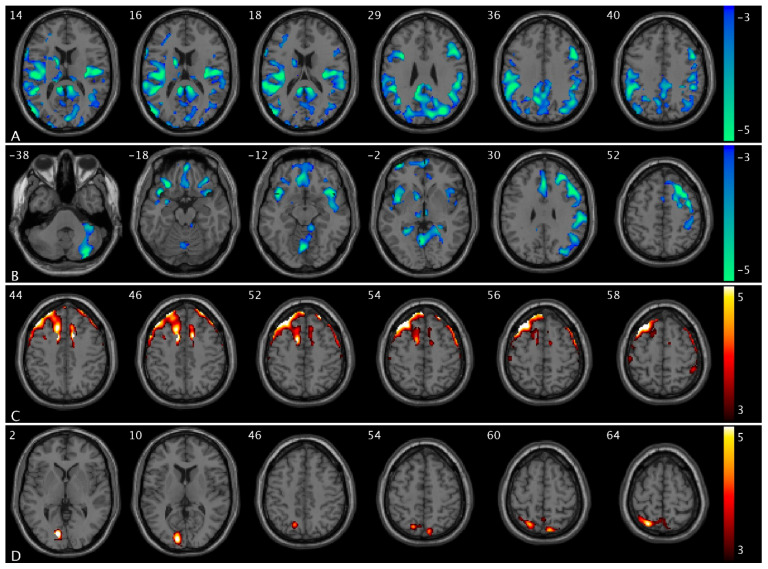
Statistical maps overlaid on T1-weighted images showing altered CBF in OAB participants compared to controls in the low- and high-urge states. (A) Decreased CBF the Rolandic extending to inferior frontal, precuneus, supramarginal, and angular extending to inferior parietal cortex, Heschl extending to superior and middle temporal cortex, calcarine and cuneus extending to middle and superior occipital cortex, insula, thalamus, caudate, PCC, middle cingulate cortex (within the same slice range as in Figure 1B) in OAB participants in the low-urge state; (B) decreased CBF in the Rolandic, rectus, precentral extending to middle, superior, inferior, and orbital frontal cortex, angular/supramarginal, Heschl extending to superior temporal cortex, vermis extending to cerebellum, insula, thalamus, caudate, pallidum, amygdala, and anterior cingulate cortex (ACC) regions in OAB participants in the high-urge state; (C) increased CBF in the supplementary motor extending to superior frontal and middle frontal cortex (within the same slice range as in Figure 1A) in OAB participants in the low-urge state; and (D) increased CBF in the inferior occipital regions in OAB participants in the high-urge state compared to healthy controls. Additional decreased CBF and increased CBF regions in OAB participants in the low-urge state are shown in Figures S1A and S1B, respectively. The numbers in the top left corner of the MRI images indicate the z-coordinate (in mm) in the MNI space. The color bars represent t-values.
